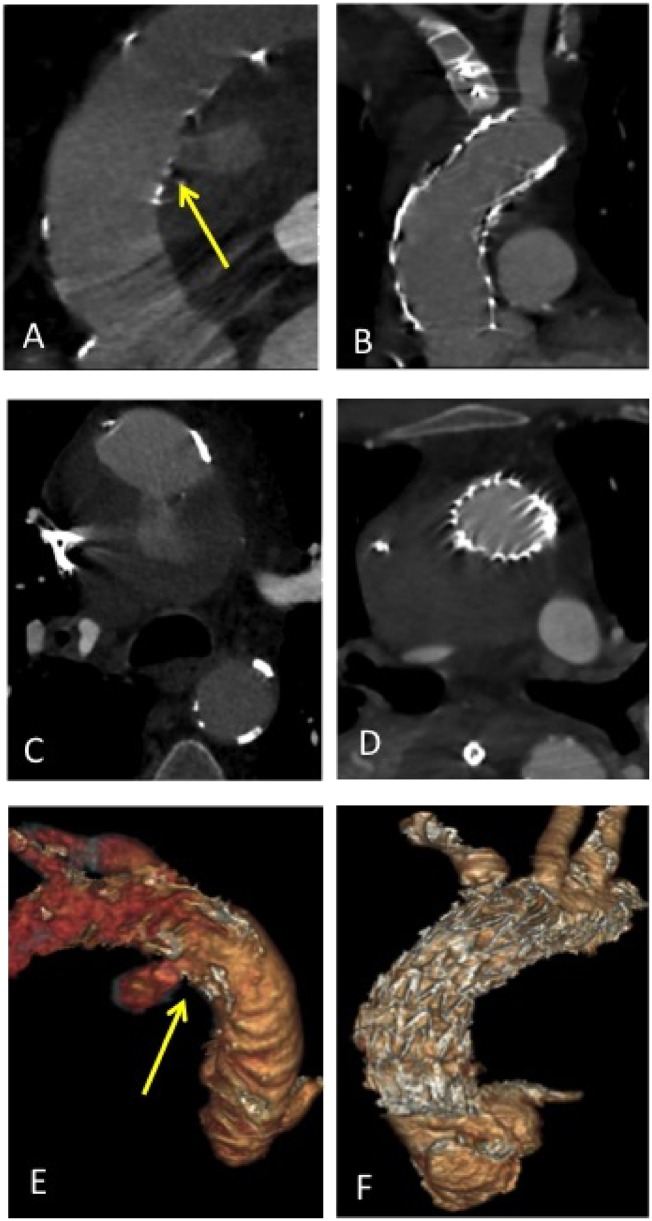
(*A–D*) Initial computed tomography (*A* and *C*) and post-operative implantation (*B* and *D*). (*E* and *F*) Three-dimensional volume rendering reconstruction of initial computed tomography (*E*) and post-operative computed tomography (*F*). (*A*, *C*, and *E*) Local aortic plaque rupture of the ascending aorta with active contrast leakage. The leak is located close to the innominate artery (yellow arrow). (*B* and *D*) Post-operative computed tomography with off the shelf thoracic stent graft Conformable GORE^®^ TAG^®^ Thoracic Endoprothesis (WL Gore & associates, Flagstaff, AZ, USA) with rupture exclusion. (*F*) The shelf thoracic stent graft Conformable GORE^®^ TAG^®^ Thoracic Endoprothesis (WL Gore & associates, Flagstaff, AZ, USA) covering the IA.
